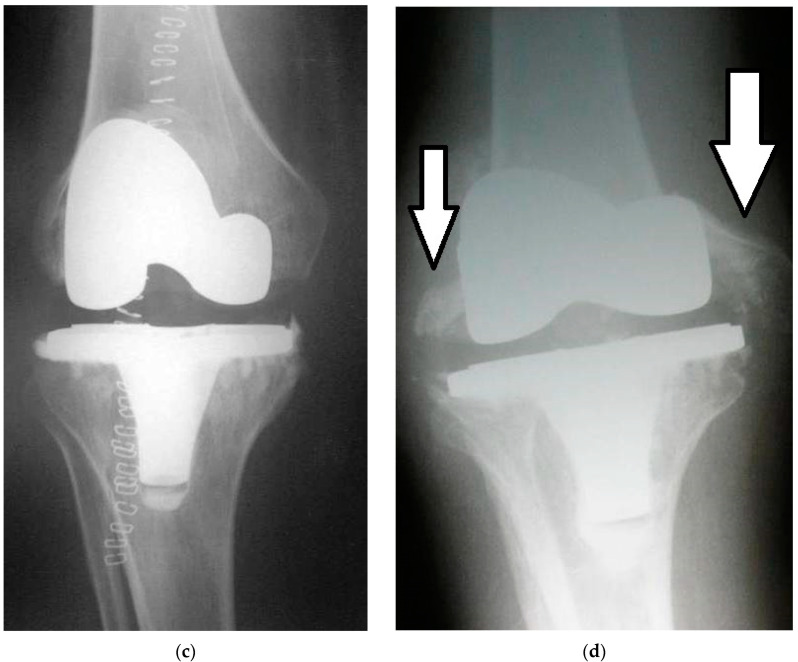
(a–d). *Staphyloccocus aureus* infection of a primary total knee arthroplasty (TKA): (a) Preoperative radiograph showing severe knee osteoarthritis; (b) Intraoperative image showing severe joint degeneration caused by osteoarthritis; (c) Immediate postoperative radiograph showing the implanted primary TKA (posterior stabilised cemented); (d) Radiographic image 5 months postoperatively showing severe osteolysis of the femoral condyles (arrows) due to periprosthetic joint infection (PJI). This patient required a two-stage revision arthroplasty in order to cure the infection.
